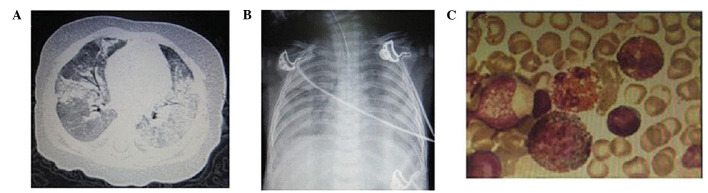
Clinical examination. (A) Chest computed tomography scan revealing extensive consolidation on both sides of the lung; (B) Chest X-ray revealing acute respiratory distress syndrome; (C) Bone marrow examination revealing additional eosinophils.
